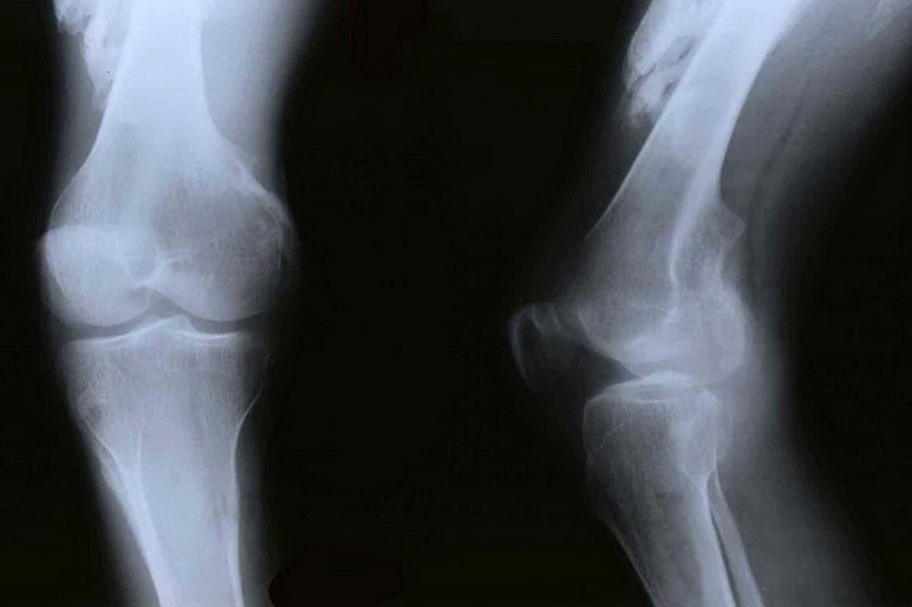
Lower limb radiographs showed ectopic ossification in the quadriceps muscle.
